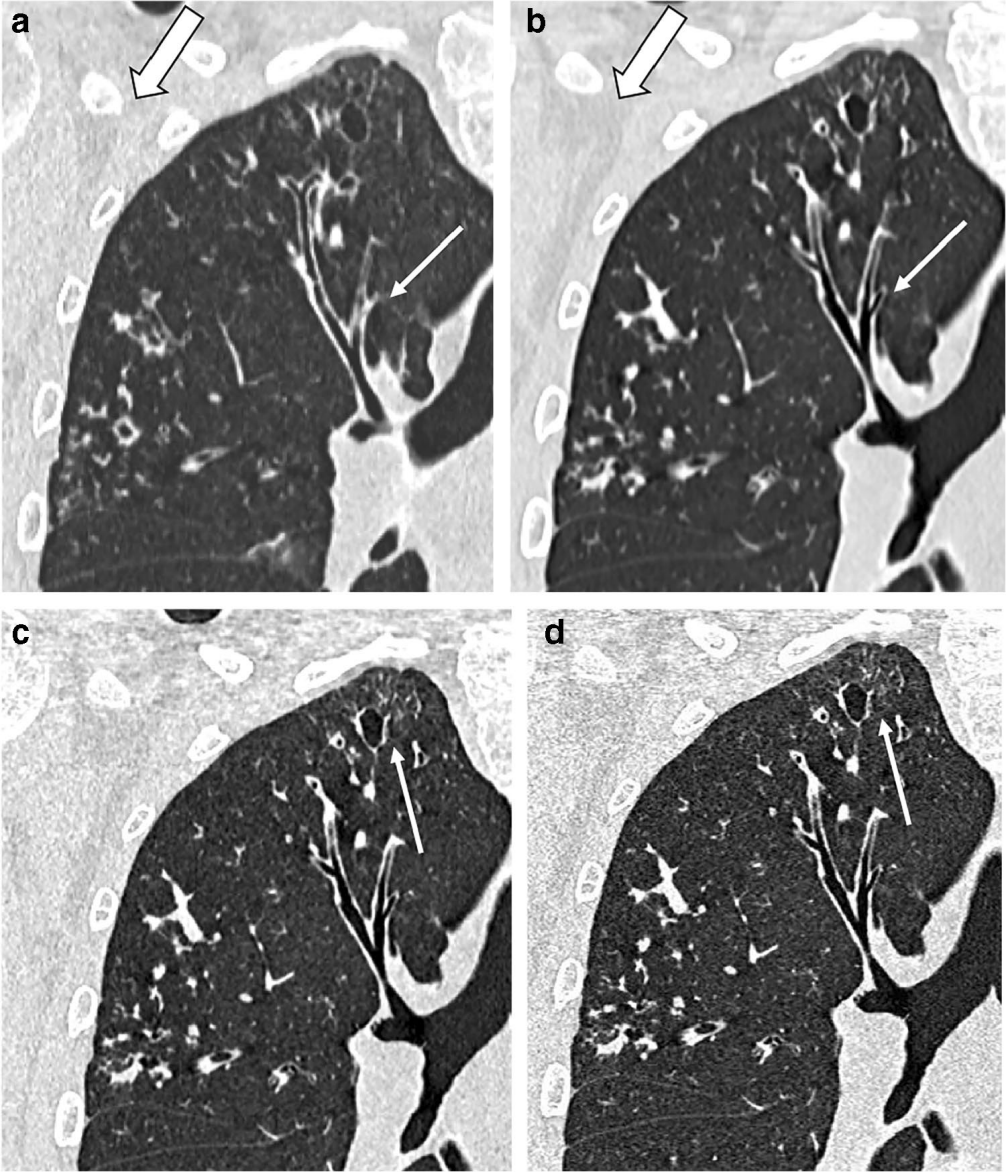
Photon-counting detector CT (PCD-CT) and conventional energy integrating detector CT (EID-CT) scanning in a 20-year-old man with cystic fibrosis. a–d Coronal detail of end-inspiratory CT using conventional EID-CT (a) and PCD-CT (b–d). Images (a) and (b) have slice thickness of 1 mm, and images (c) and (d), 0.6 mm and 0.2 mm, respectively. All four images have similar kernels including iterative reconstruction. Comparison of images (a) and (b) shows a clear reduction of image noise (reduced granularity in the axillary region, *thick arrow*) and sharper definition of bronchial wall and cystic bronchiectasis in the PCD-CT (*thin arrow*). In images (c) and (d), the increased resolution allows sharper details of the most peripheral structures (*thin arrow*)
